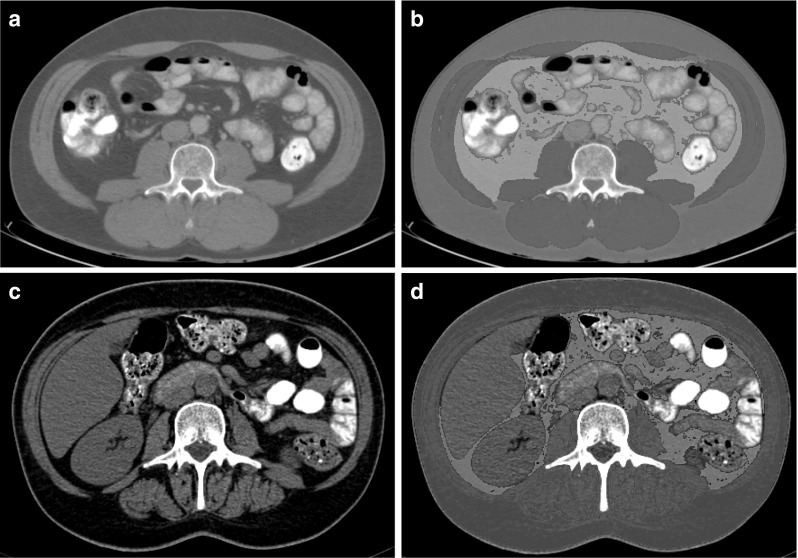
Computed tomography (CT) images showing the measurement of skeletal muscle and different types of adipose tissue at the level of the third lumbar vertebra (L3); a and b show the original and tagged (i.e., colored) CT images, respectively, of one included CRC survivor [man, 50 years old at CRC diagnosis, self-reported body mass index (BMI) at diagnosis: 27.6 kg/m^2^] with a relatively high skeletal muscle index (67.0 cm^2^/m^2^); area of visceral adipose tissue and intermuscular adipose tissue: 118.3 and 10.1 cm^2^, respectively; mean muscle attenuation: 35.9 Hounsfield units (HU); c and d show the original and tagged CT images, respectively, of one included CRC survivor (woman, 49 years old at CRC diagnosis, BMI at diagnosis: 21.0) with a relatively low skeletal muscle index (30.8 cm^2^/m^2^); area of visceral adipose tissue and intermuscular adipose tissue: 52.9 and 20.4 cm^2^, respectively; mean muscle attenuation: 27.2 HU. Within the tagged figure, *yellow* represents visceral adipose tissue; *blue* represents subcutaneous adipose tissue; *green* represents intermuscular adipose tissue; and *red* represents skeletal muscle. (Color figure online)
